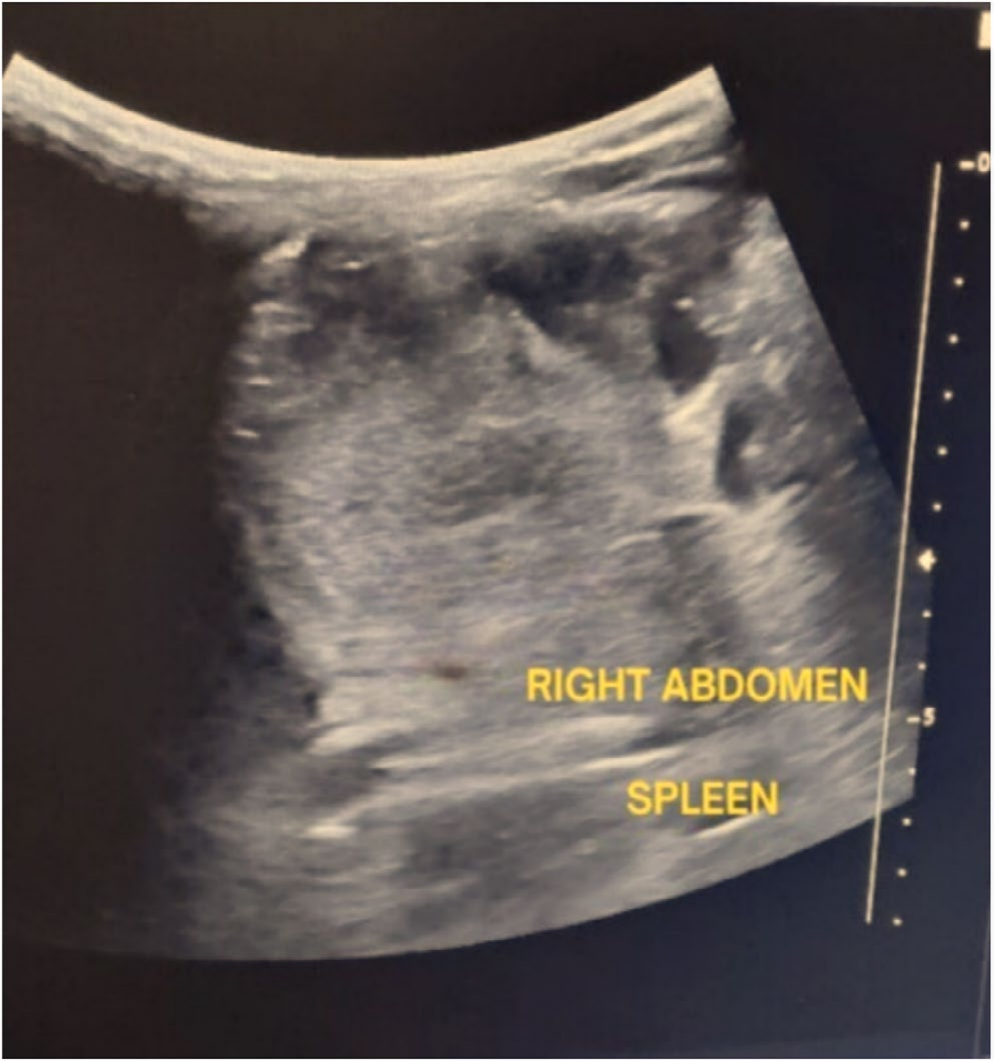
A right‐sided spleen is shown in an ultrasonography image of the right side of the abdomen as opposed to its typical anatomical location.
